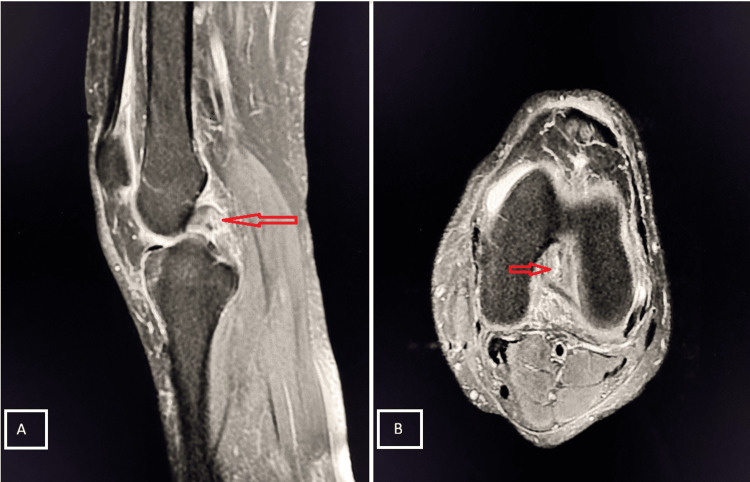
Grade III tear of the PCL Sagittal PD-FS (A) and axial (B) MRI demonstrates a hyperintense, thickened PCL with complete disruption at the mid-portion, consistent with a Grade III PCL tear (red arrows). Mild joint effusion and peri-ligamentous soft tissue edema are noted. PCL, posterior cruciate ligament; PD-FS, proton density fat-suppressed
